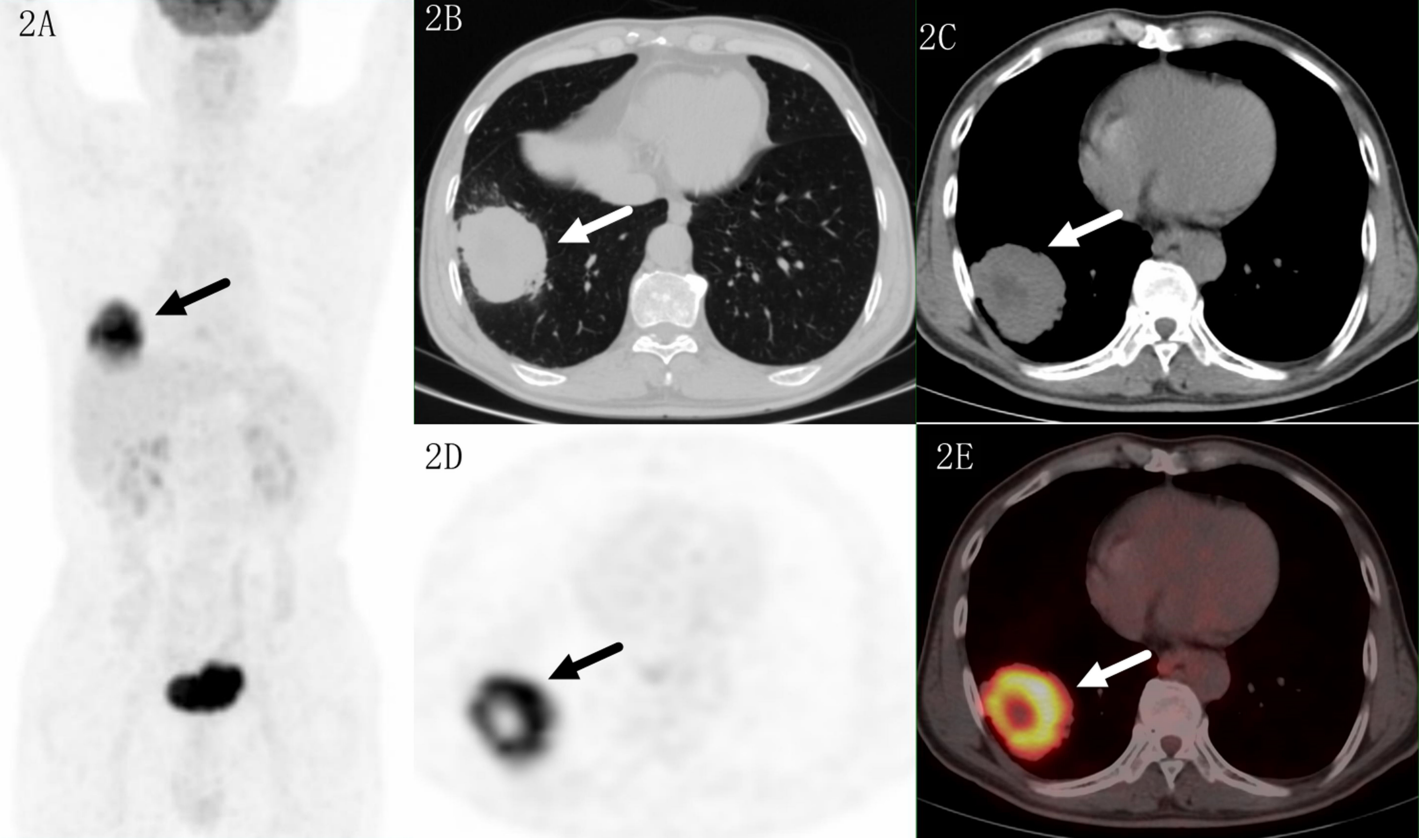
Images of a 69-year-old male patient with adenocarcinoma. PET MIP (A) showed a mass in the right lower lobe and a suspected lymph nodes in the right hilar. The lesion located in sub pleural site with a SUVmax of 16.4 on transaxial CT (B, C), PET (D) and fused (E) images. Higher uptake was seen in the proximal region which indicated malignant lesion.
